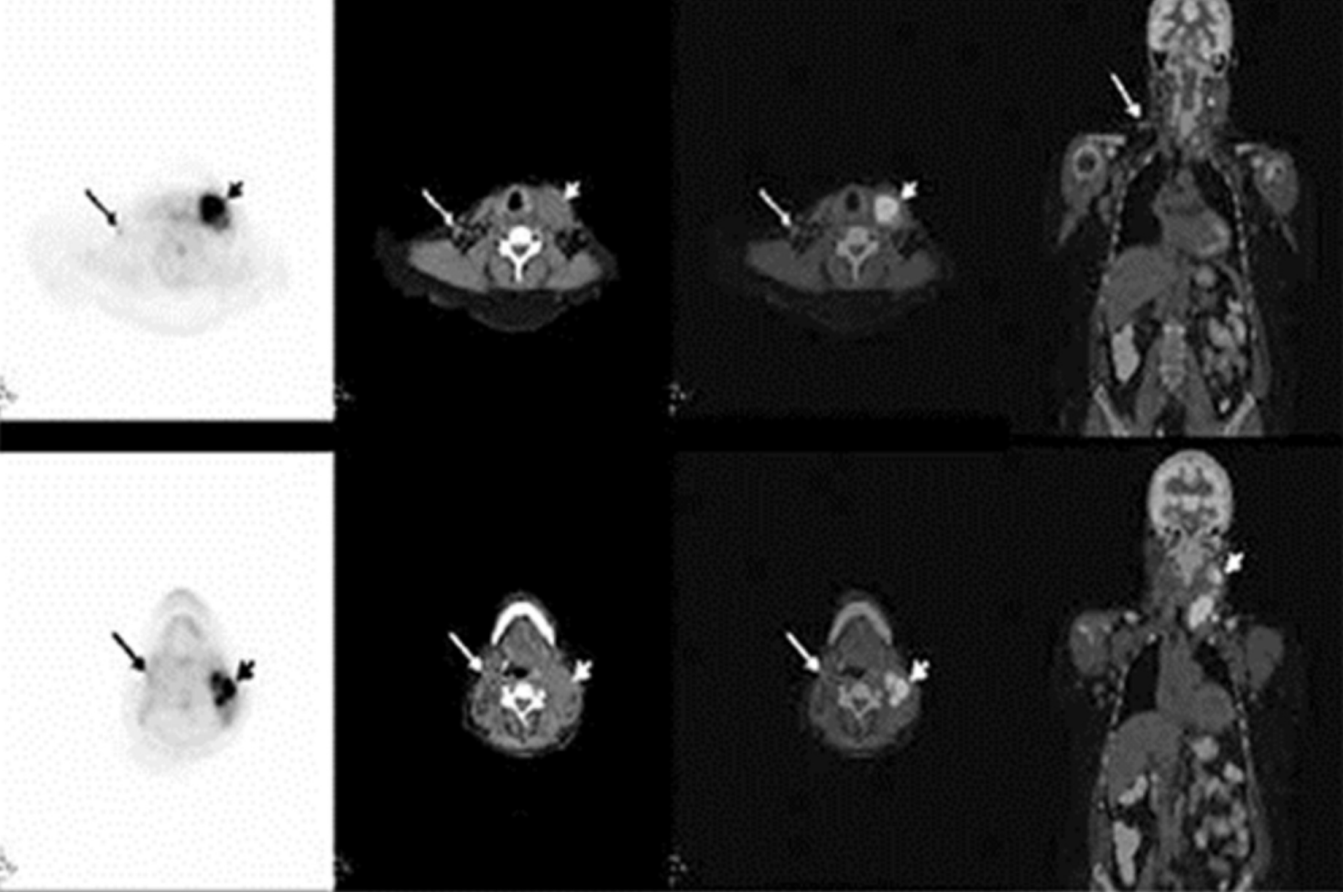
FDG-PET/CT images demonstrating intensely FDG-avid left cervical nodes suggestive of Richter transformation Axial PET, CT, and fused PET/CT images revealed left submandibular and upper-middle jugular conglomerate lymph nodes (short arrow) with intense FDG uptake (SUVmax 13), suggesting transformation. Additionally, minimally increased FDG uptake in the right jugular chain (long arrow) compatible with indolent lymphoma involvement was noted. (Reference: Yilmaz, et al. [21]).
